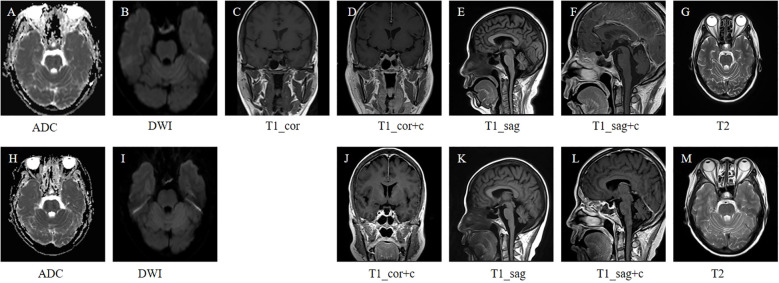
Comparative pituitary MRI scans before and after treatment. (A-G) Pre-treatment pituitary MRI revealed glandular enlargement, stalk thickening, and marked enhancement-features most consistent with an inflammatory etiology-with preservation of the neurohypophyseal bright spot (2024.10.28); (H-M) Pituitary MRI after two treatment cycles revealed a reduction in pituitary volume and mild thickening of the pituitary stalk, indicating improvement compared to the previous scan (2025.1.13). ADC, Apparent Diffusion Coefficient; DWI, Diffusion-Weighted Imaging: T1_cor, T1-weighted coronal image; T1_sag, T1- weighted sagittal MRI; T1_sag+c, T1-weighted contrast-enhanced sagittal; T2, Transverse relaxation time.
